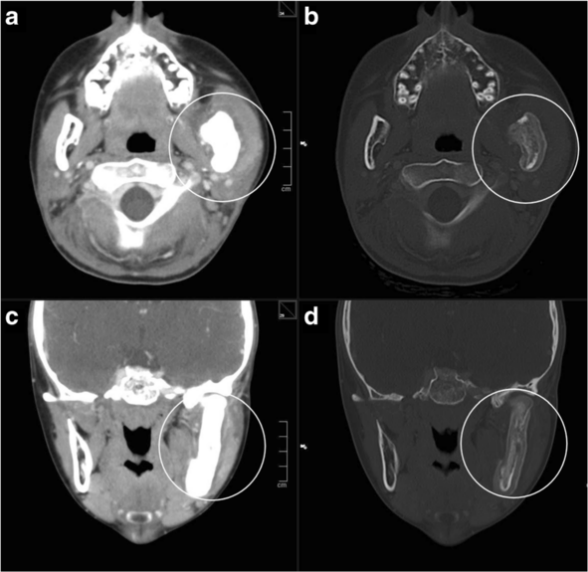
a, c The CT reveals an extensive thickening of the left mandible with contrast agent enhancing soft tissue mass (*circles*). b, d Destruction of the cortex of the mandible and massive periostal reaction. Similar changes can also be found in the posterior aspect of the cervical spine (*circles*)
